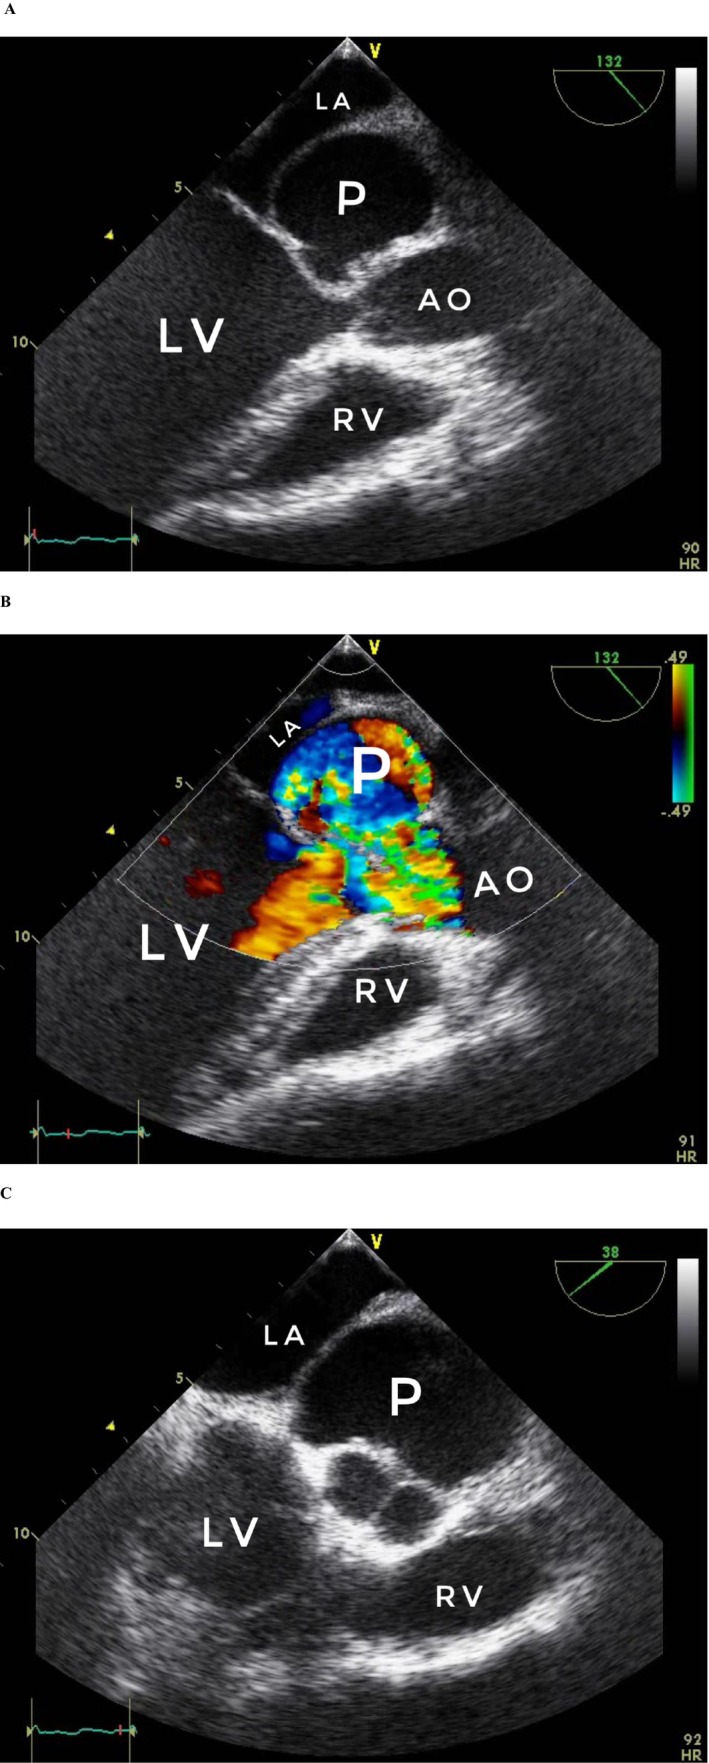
(A) Long axis view. (B) Long axis view. (C) Short axis view. Trans‐thoracic echocardiography short and long axis views. AO, aorta; LA, left atrium; L, left ventricle; P, pseudoaneurysm of the mitral‐aortic intervalvular fibrosa; RV, right ventricle.
